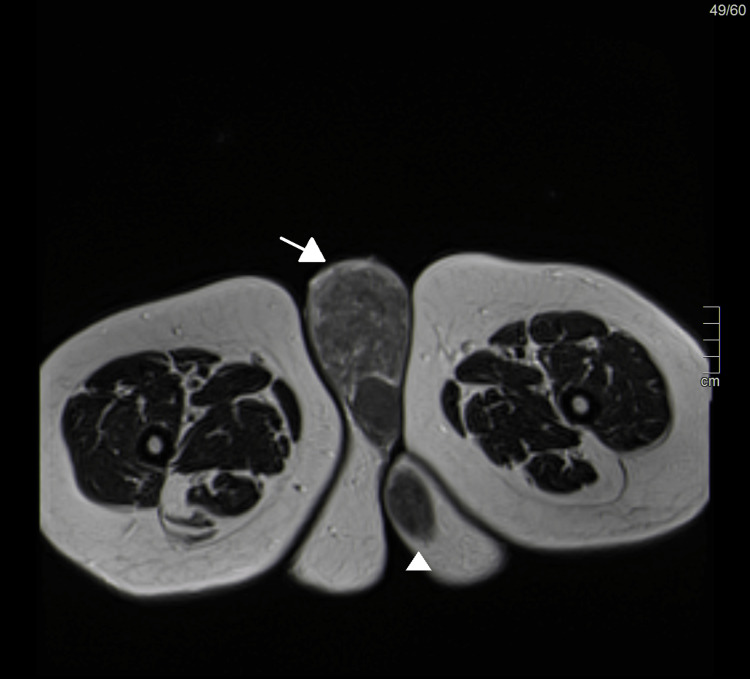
T2-weighted magnetic resonance imaging showing hypointense vulvar (arrow) and perineal masses (arrowhead)
